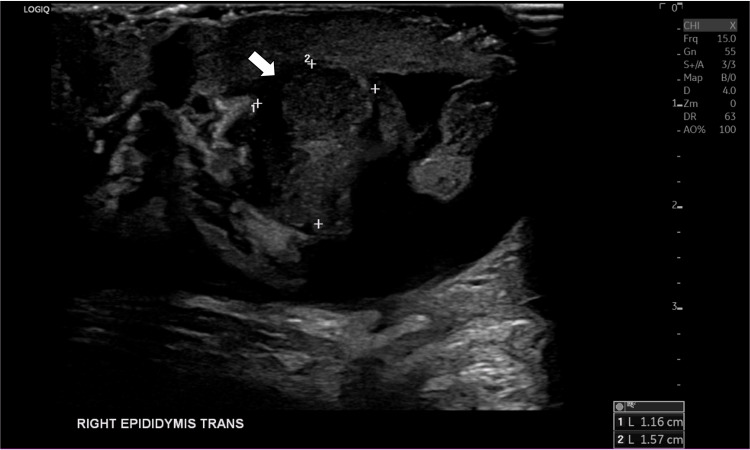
Scrotal ultrasound showed a concurrent right scrotal fluid collection and right epididymo-orchitis (arrow)
